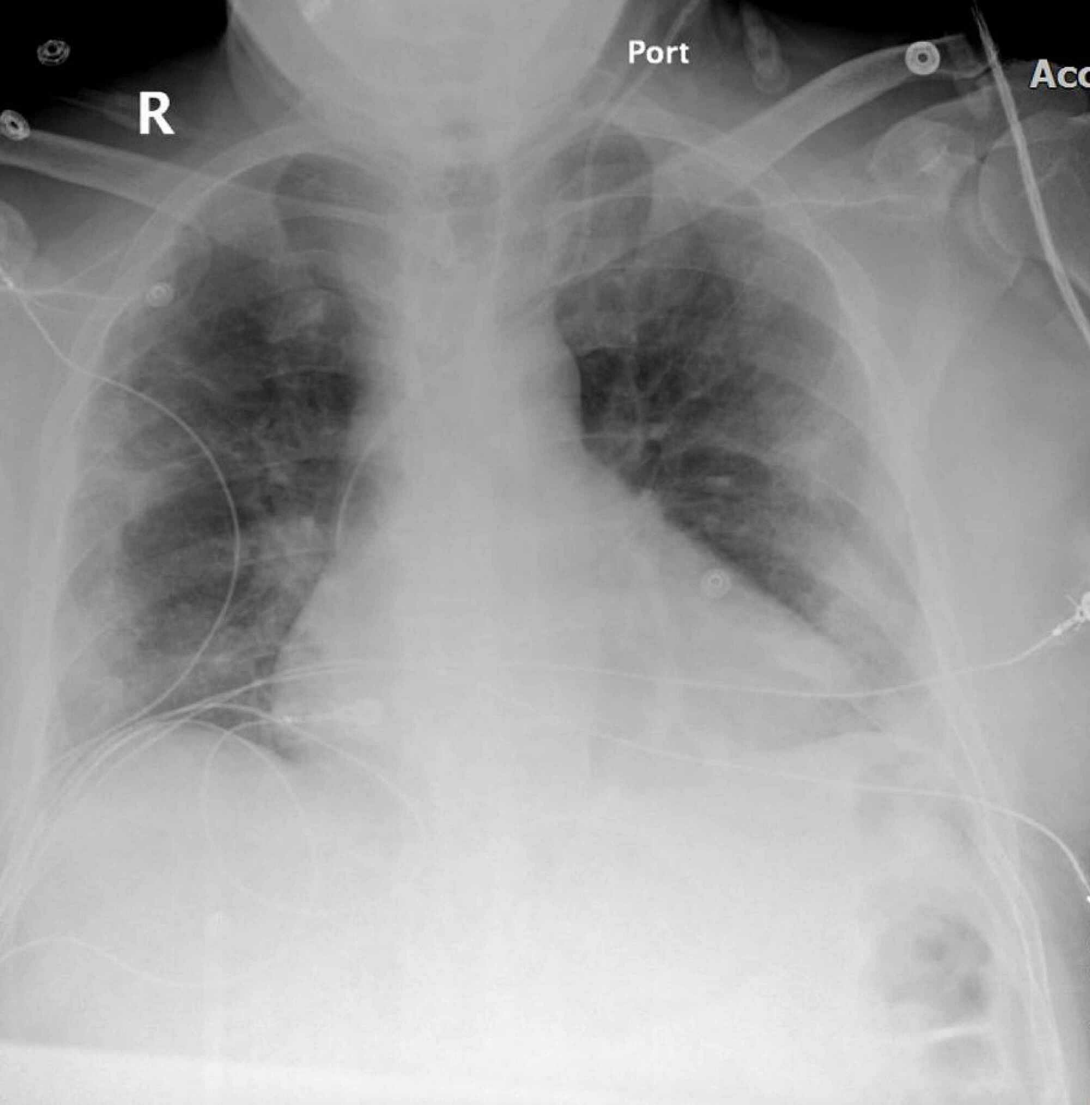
Chest X-ray showing cardiomegaly.
